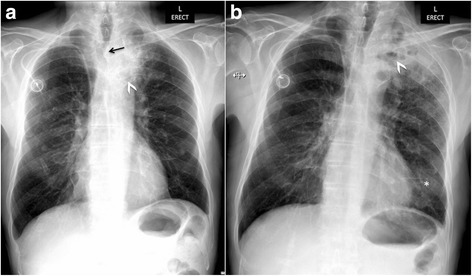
(a) Initial chest x-ray reveals an apical left lung partially excavated opacity (*arrowhead*) associated with a silhouette sign of the upper left mediastinum, consistent with secondary tumor infection. Note that owing to mass effect, there is right-sided tracheal deviation (*arrow*). (b) A control chest x-ray obtained 10 days later shows an unfavorable evolution as seen by an increased infectious tumor focus, progression of the excavated components (*arrowhead*), and occurrence of lingular opacity (*asterisk*)
